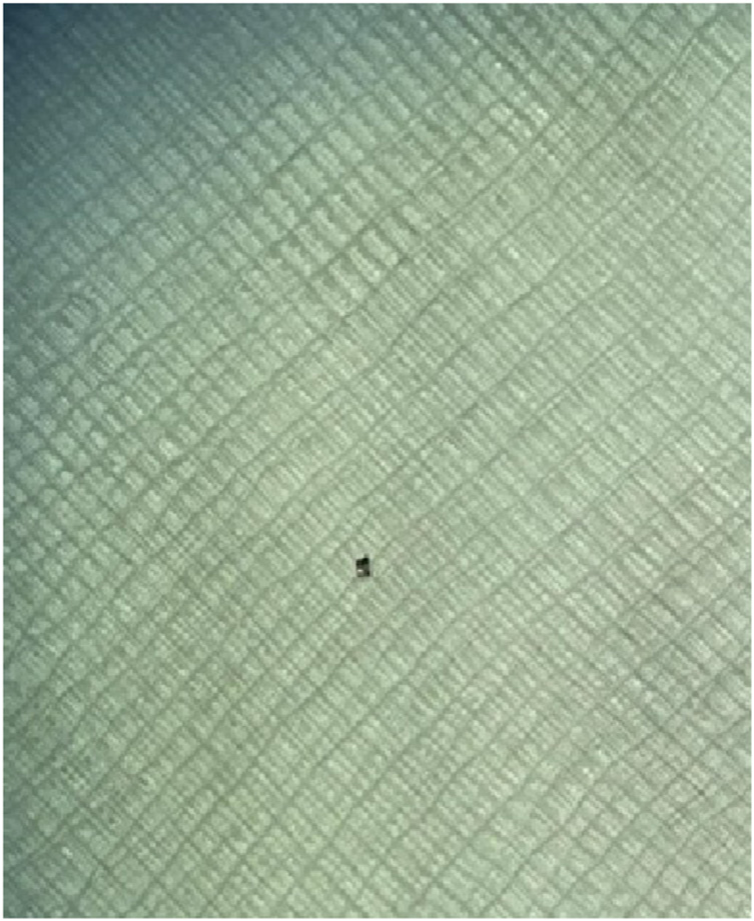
Retrieved spline electrode. As noted in the text, the Vizigo steerable sheath was advanced immediately adjacent to the electrode, confirmed by correlating fluoroscopic imaging, intracardiac echocardiography, and the electroanatomic map. A 60 cc syringe was then attached to the side port of the sheath, and manual suction was used to retrieve the electrode.
